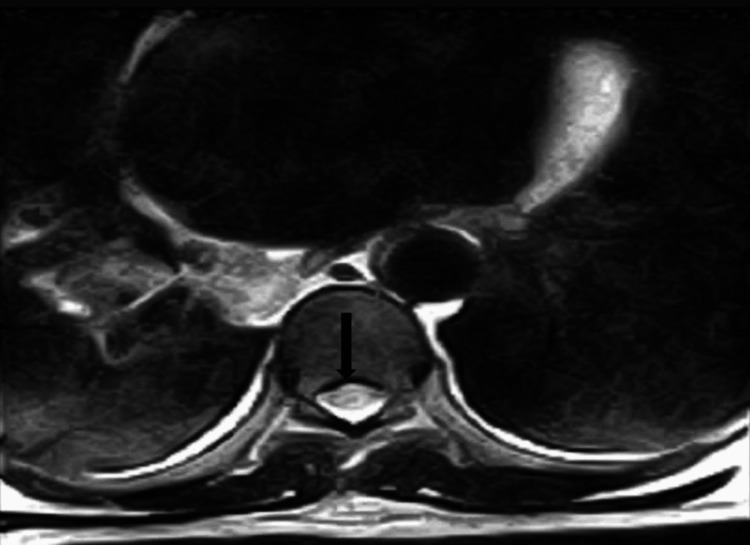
The axial T2-weighted thoracic MRI documented ring enhancement with a central low signal intensity intramedullary lesion.
